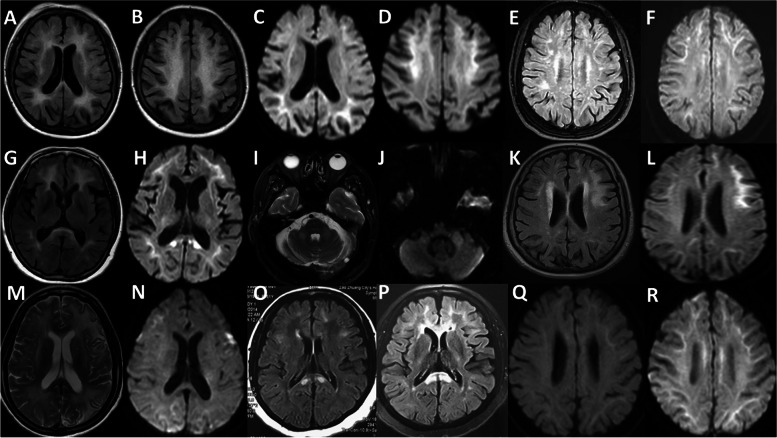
A-D F/64, widespread leukoencephalopathy with frontal lobe predominance and corticomedullary junction DWI high intensity, involving corpus callosum and external capsule. E–F F/55, patchy frontal lobe leukoencephalopathy with corticomedullary junction DWI high intensity. G-H F/59, frontal lobe and external capsule DWI high intensity with splenium of corpus callosum lesions. I-J M/61, symmetrical lesions on brachium pontis and DWI high intensity. K-L F/54, asymmetrical distribution of frontal lobe leukoencephalopathy. M–N F/45, widespread leukoencephalopathy with grey matter involvement, left predominance. O-P F/54, progression of leukoencephalopathy after 4 years follow-up. Q-R F/51, extensive increase of corticomedullary junction DWI high signal after 2 months follow up, while no change in FLAIR sequence. (FLAIR: A, B, E, G, K, O, P; T2-weighted: I, M; DWI: C, D, F, H, J, L, N, Q, R)
